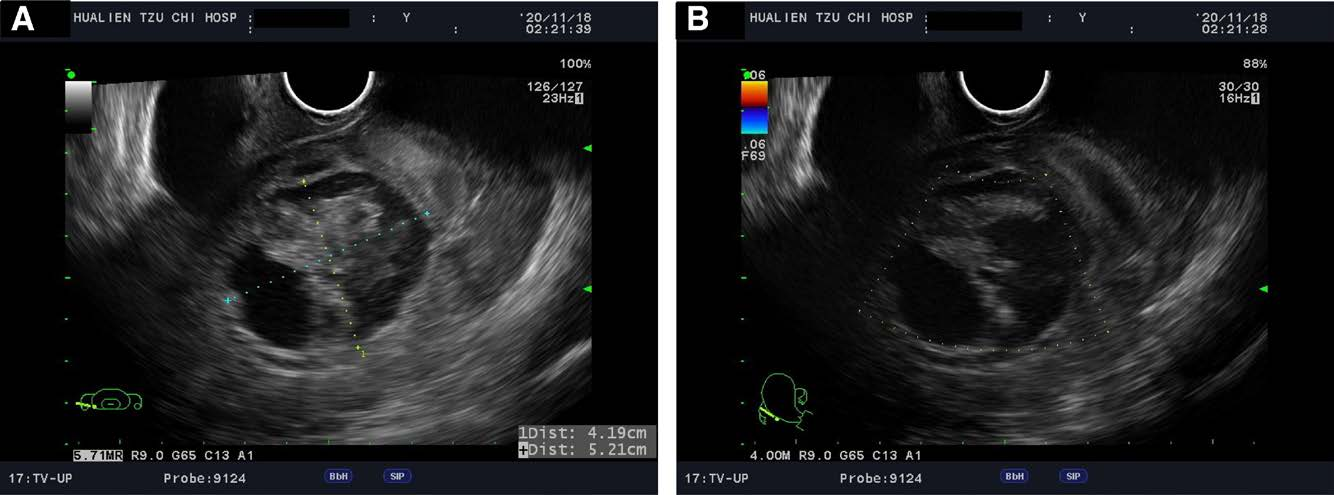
(A) Transvaginal ultrasound showed a heterogeneous right adnexal mass about 5 × 6 × 5 cm in size with soft tissue and fluid in content. (B) Absence of arterial flow on color Doppler within the adnexal tumor.
